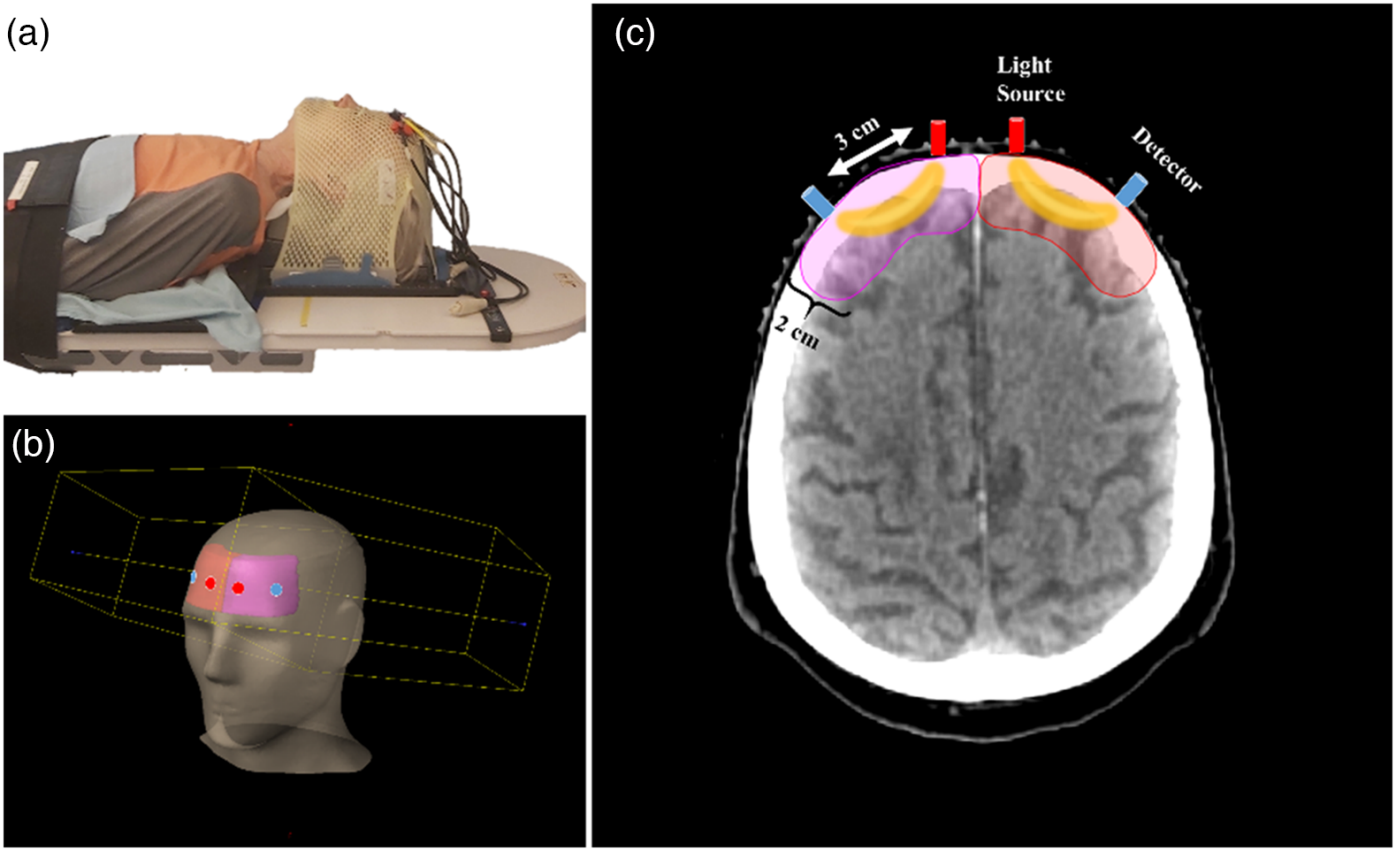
During the treatment, patients wear a plastic mesh mask to ensure the correct patient positioning to make the irradiation spatially accurate and to avoid patient movement. The mask is molded to perfectly fit the patient’s head shape. Multiple fibers can be easily attached to the mask for the fNIRS measurements. The fiber output tip (diameter of 2.5 mm) with a 90-deg bend are attached to forehead on left (NIRS ch1) and right (NIRS ch2) side using 3D printed fiber clips that are glued on the mask. (a) Patient is in the treatment position in the medical LINAC (TrueBeam, Varian Medical Systems), when optical fiber tips are attached perpendicular toward the brain at (b) source–detector distance of 3 cm and (c) the corresponding CT image.
